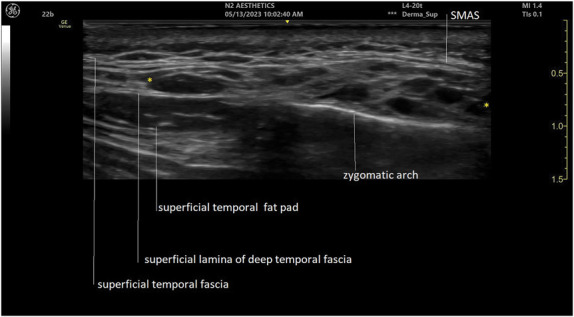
Ultrasound (grayscale, transverse view) of the zygomatic and temple area showing filler material injected in the deep fat of the lateral SOOF shifts into the deep interfascial plane of the temple. Hyaluronic acid deposits in between yellow markers (GE venue Go 20 MHz). SOOF, suborbicularis oculi fat.
